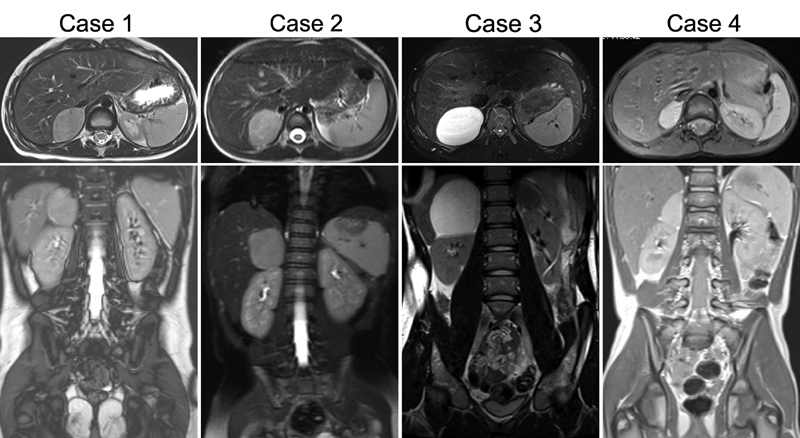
Magnetic resonance imaging of all four patients. None of them presented with image-defined risk factors.
